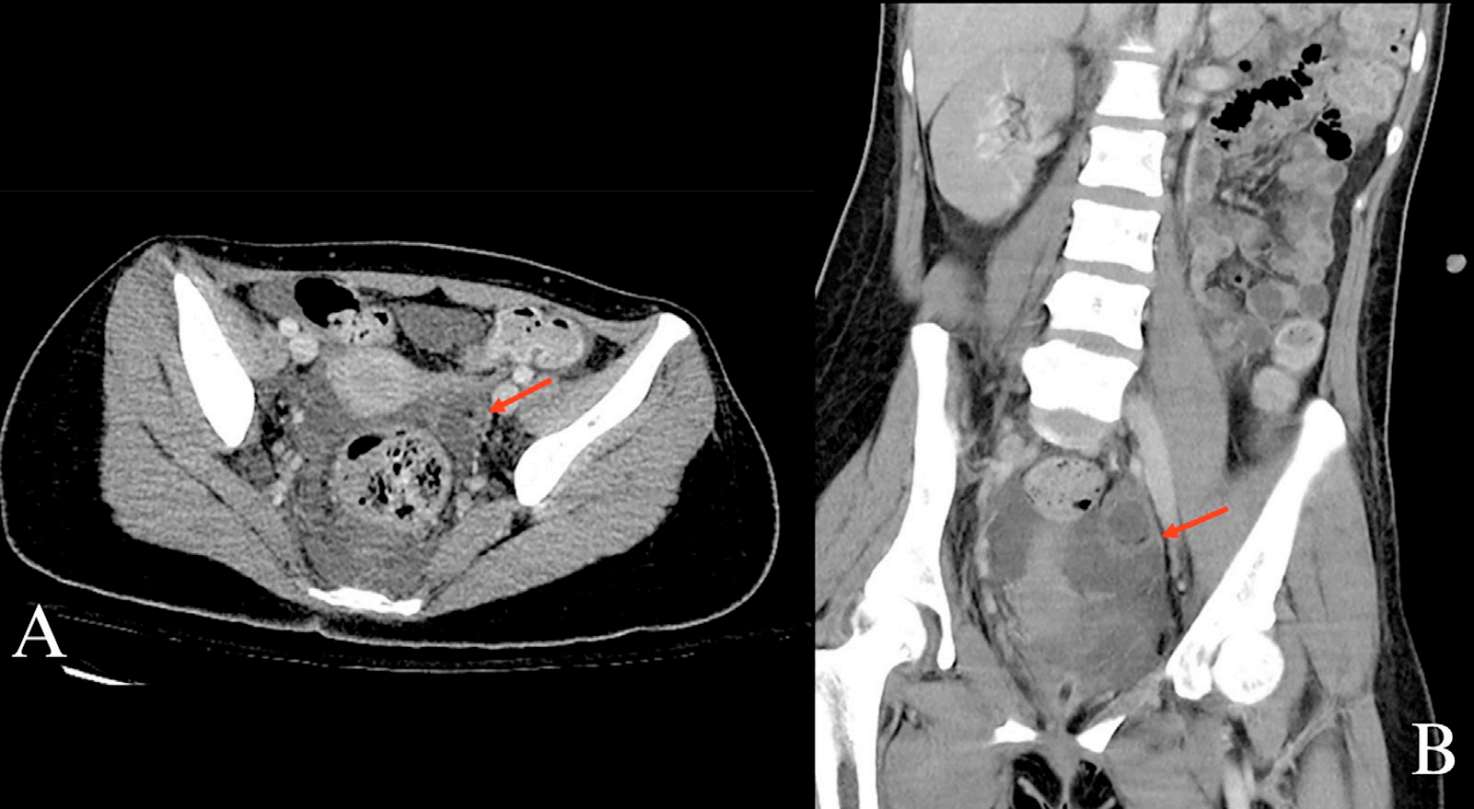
(A) Axial computed tomography (CT) of the abdomen and pelvis with contrast shows a left ovarian lesion (red arrow) measuring up to 2.7cm with a tiny focus of macroscopic fat in keeping with an ovarian teratoma. (B) Coronal CT of the abdomen and pelvis with contrast shows the same lesion (red arrow) as described above.
